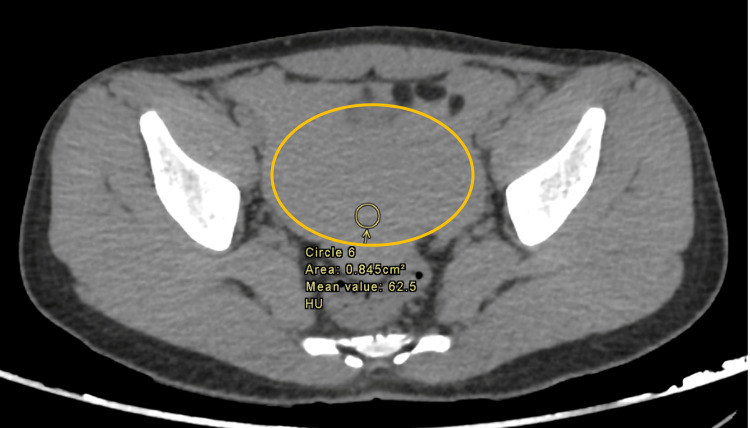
Abdominal CT scan (precontrast) demonstrating mild-moderate intra-abdominal hemoperitoneum (circle)
